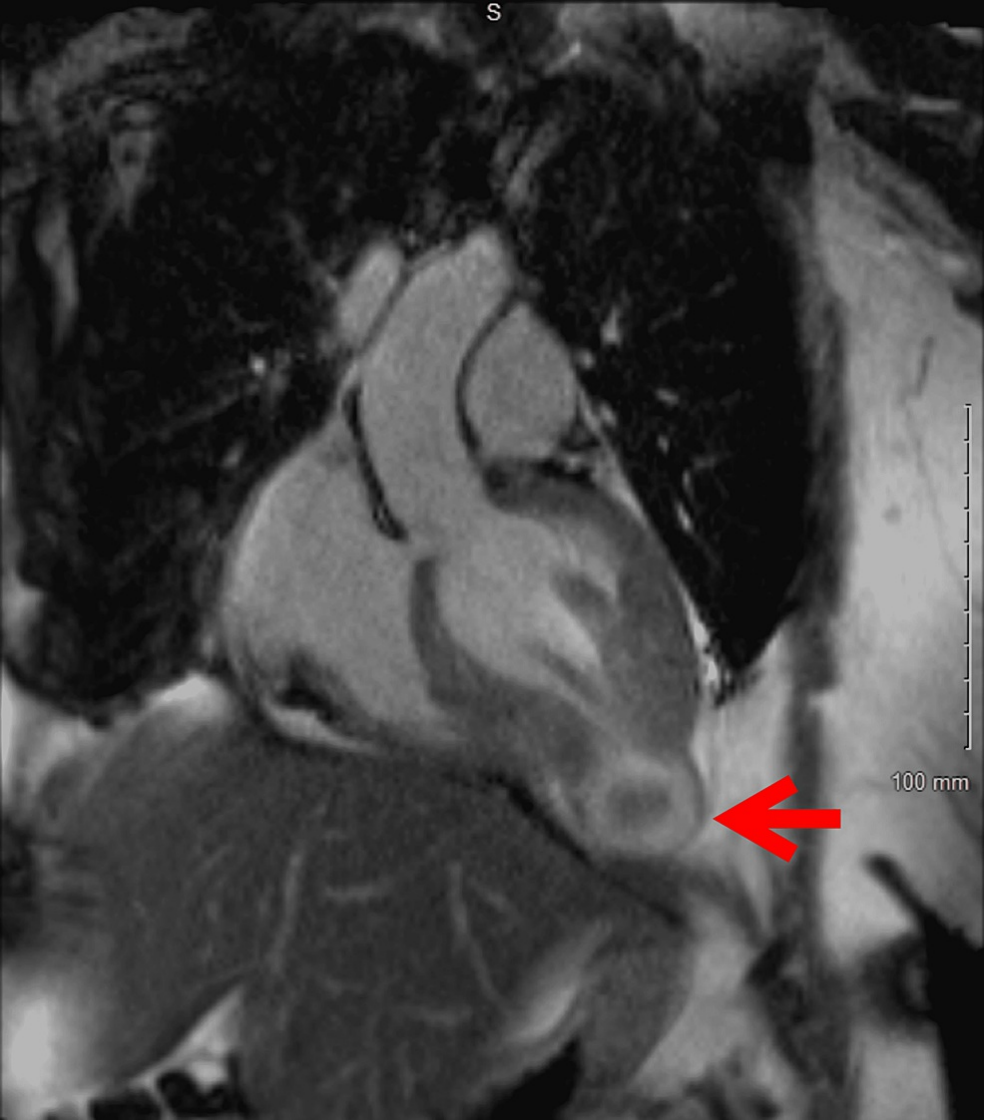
Cardiac MRI vertical long-axis view showing apical hypertrophy and an apical aneurysm A 21 x 14 mm thrombus is evident in the aneurysm, indicated with the red arrow.
